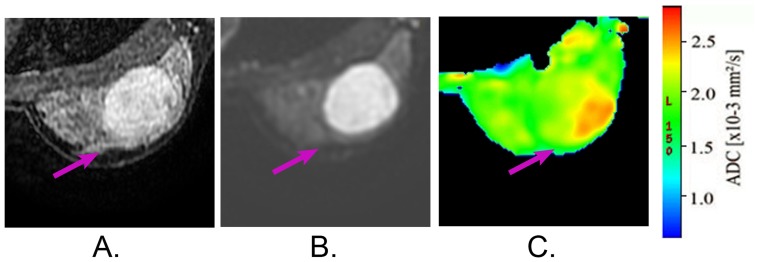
A sample image of fibroadenoma for a 28 year woman. (a) Raw dynamic contrast-enhanced MR image on lesion, which exhibits high signal intensity. The mass-like enhancement area is marked by purple arrow and the lesion; (b) Raw Diffusion-weighted MR image (b = 800 s/mm^2^); (c) Calculated ADC map from (b). Lesion area exhibits with light green (pointed in purple arrow), implying a high ADC value. ADC measured in this lesion is 1.91×10^−3^ s/mm^2^.
